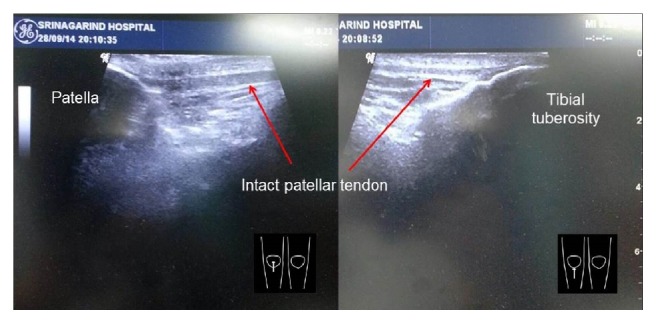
Under high frequency transducer (8 MHz linear transducer), the longitudinal scan showed multiple, parallel echogenic lines of the patellar tendon between the lower pole of the patella and the tibial tuberosity (arrowed). This ultrasound finding confirmed intact patellar tendon.
